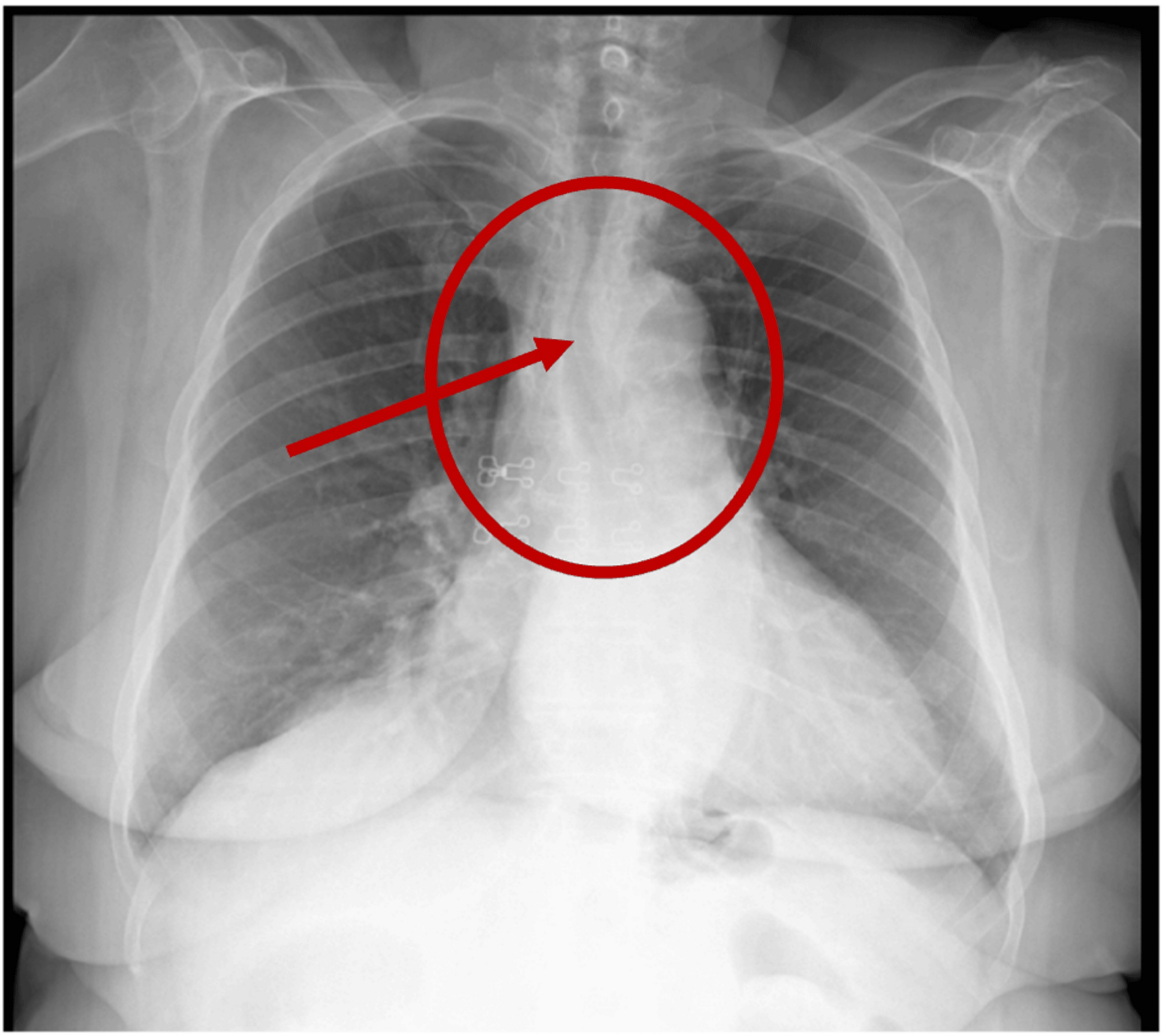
Chest X-ray showing mediastinal widening (red circle) and tracheal deviation (red arrow)
